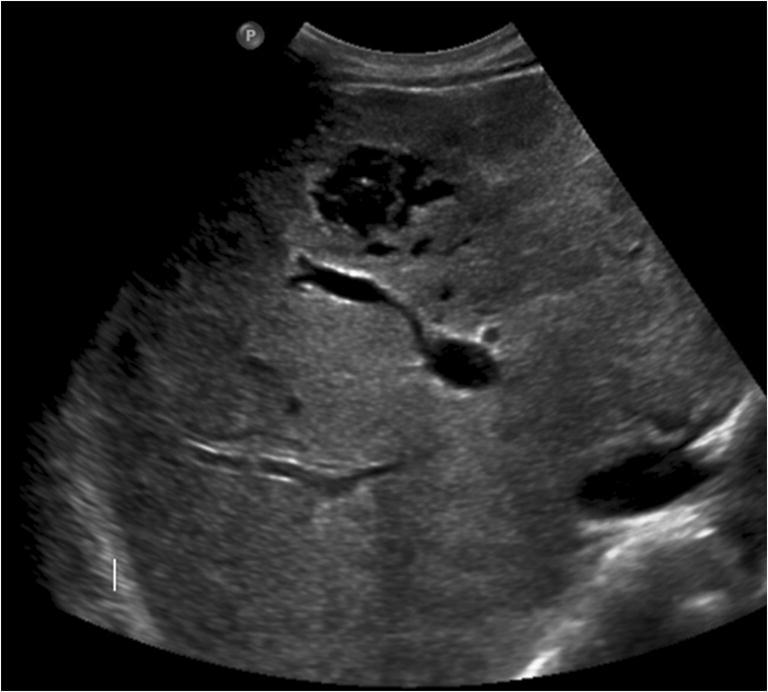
Ultrasound: multiple focal liver lesions appearing markedly inhomogeneous. Same lesions show fluid component
